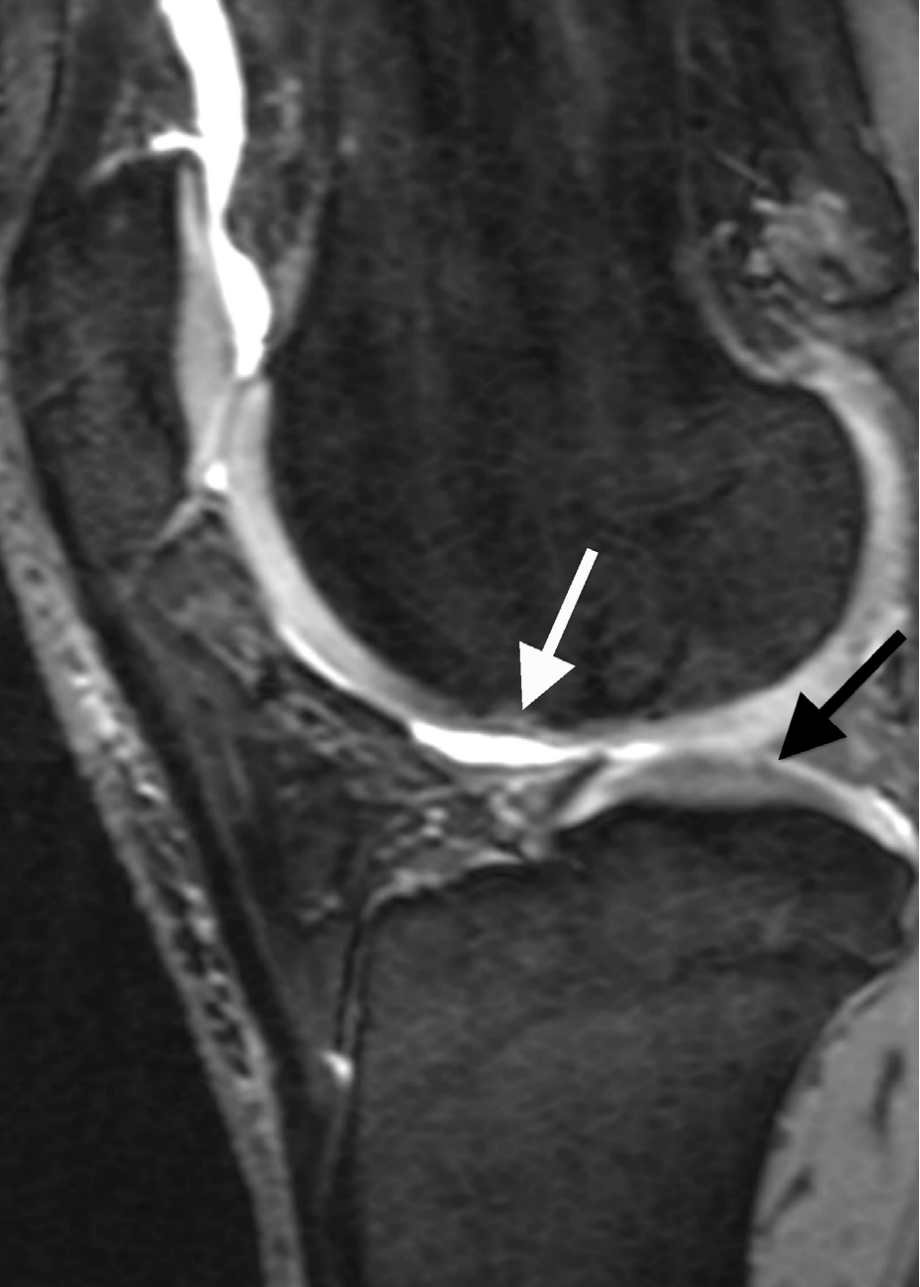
Sagittal PD-weighted TSE MRI of a 41-year-old male. A defect of more than 50% of the cartilage thickness at the medial femoral condyle is visible (white arrow). This finding is defined as grade 3 disease. A grade 2 disorder as a superficial fibrillation or erosion composing less than 50% of the cartilage was noticed at the tibial medial plateau (black arrow).
